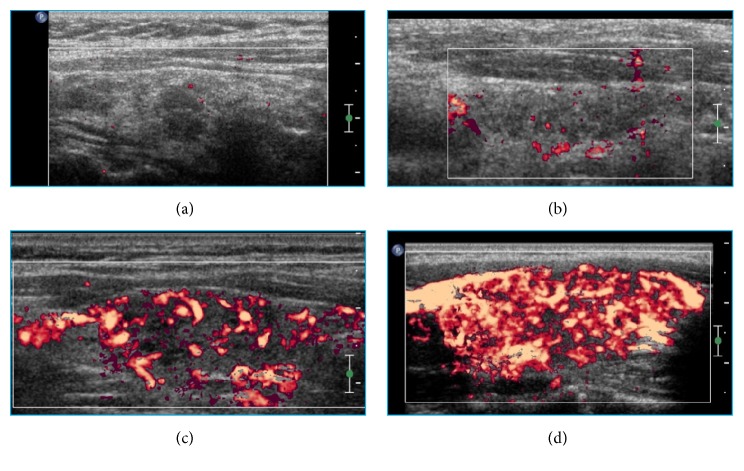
Color Doppler images. Parts (a), (b), (c), and (d) correspond to vascularization patterns 0, I, II, and III of the thyroid parenchyma, respectively.
